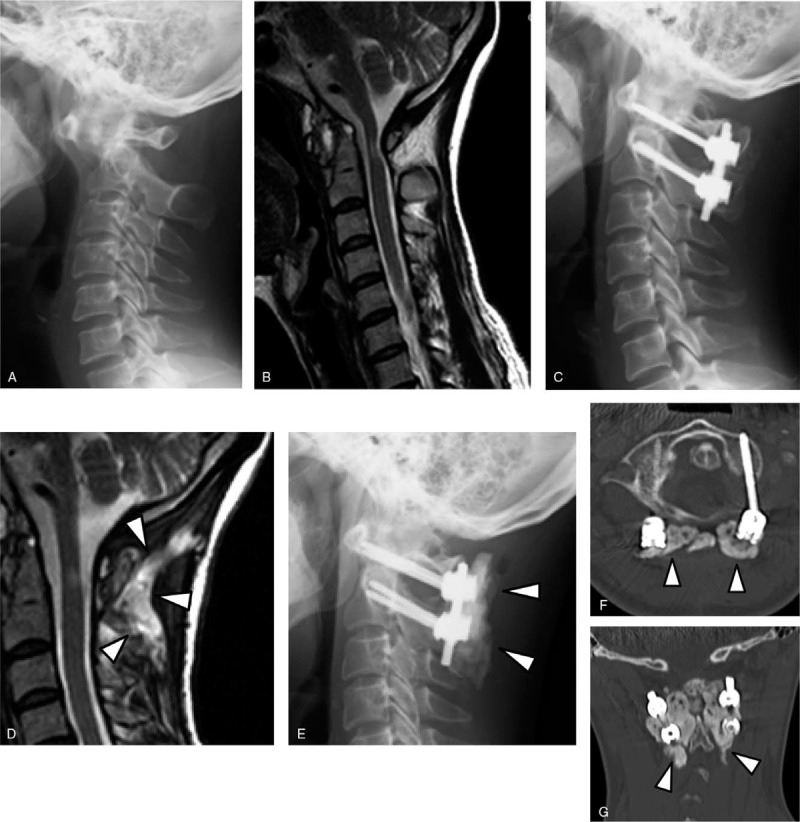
Case 1: (A) Preoperative lateral view radiograph. (B) Preoperative mid-sagittal T2-weighted MRI. (C) Postoperative lateral view radiograph. (D) Mid-sagittal T2-weighted MRI taken after the occurrence of deep SSI, revealing abscess formation in the subfascial space (*arrowhead*s). (E) Lateral view radiograph recorded following the procedure for the treatment of deep SSI. ALBC covering metal implants is shown (*arrowhead*s). (F) Axial CT image and (G) coronal CT image following the procedure for the treatment of deep SSI. The images show that ALBC embedded metal implants occupy the dead space in the operated wound (*arrowhead*s). ALBC = antibiotic-loaded bone cement, CT = computed tomography, MRI = magnetic resonance imaging, SSI = surgical site infection.
